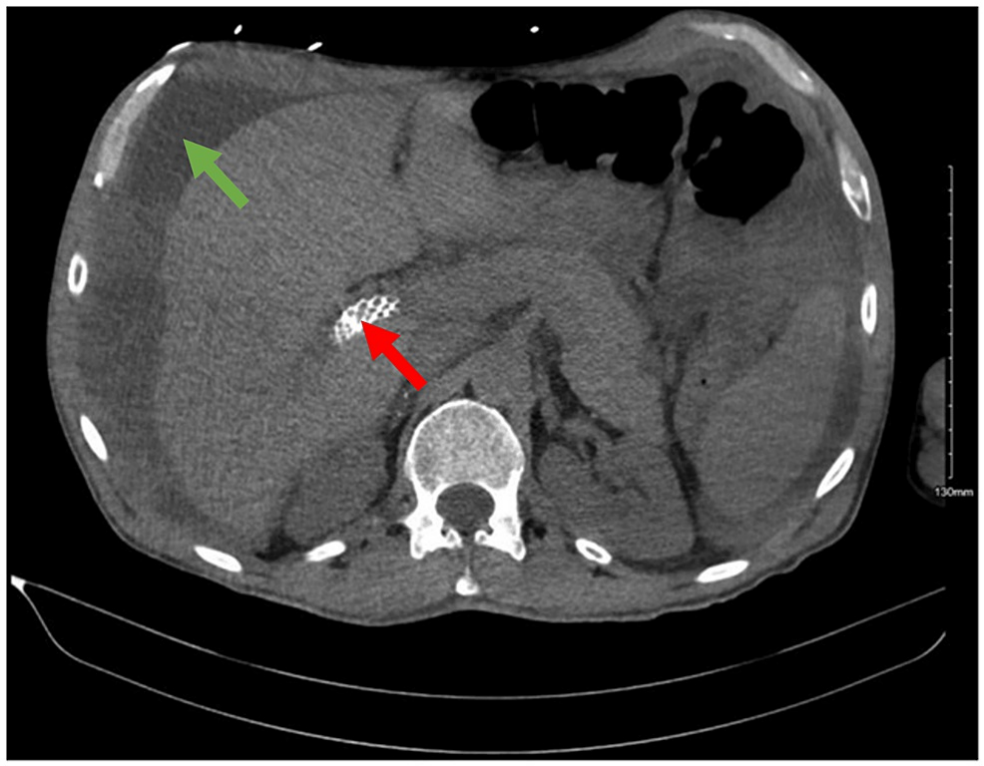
This figure depicts a CT scan of the abdomen, showing ascites (green arrow) alongside a prominently visible transjugular intrahepatic portosystemic shunt (TIPS) (red arrow).
